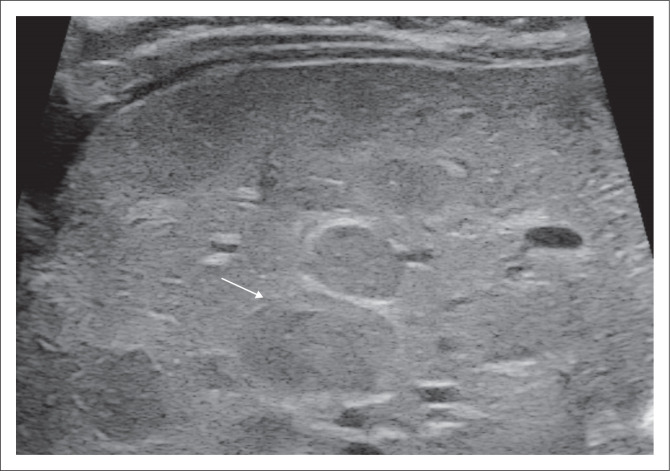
Infantile hepatic haemangioma in an 8-month-old child. Transverse grayscale ultrasound showing multiple well-defined isoechoic lesions in both the lobes of liver.
